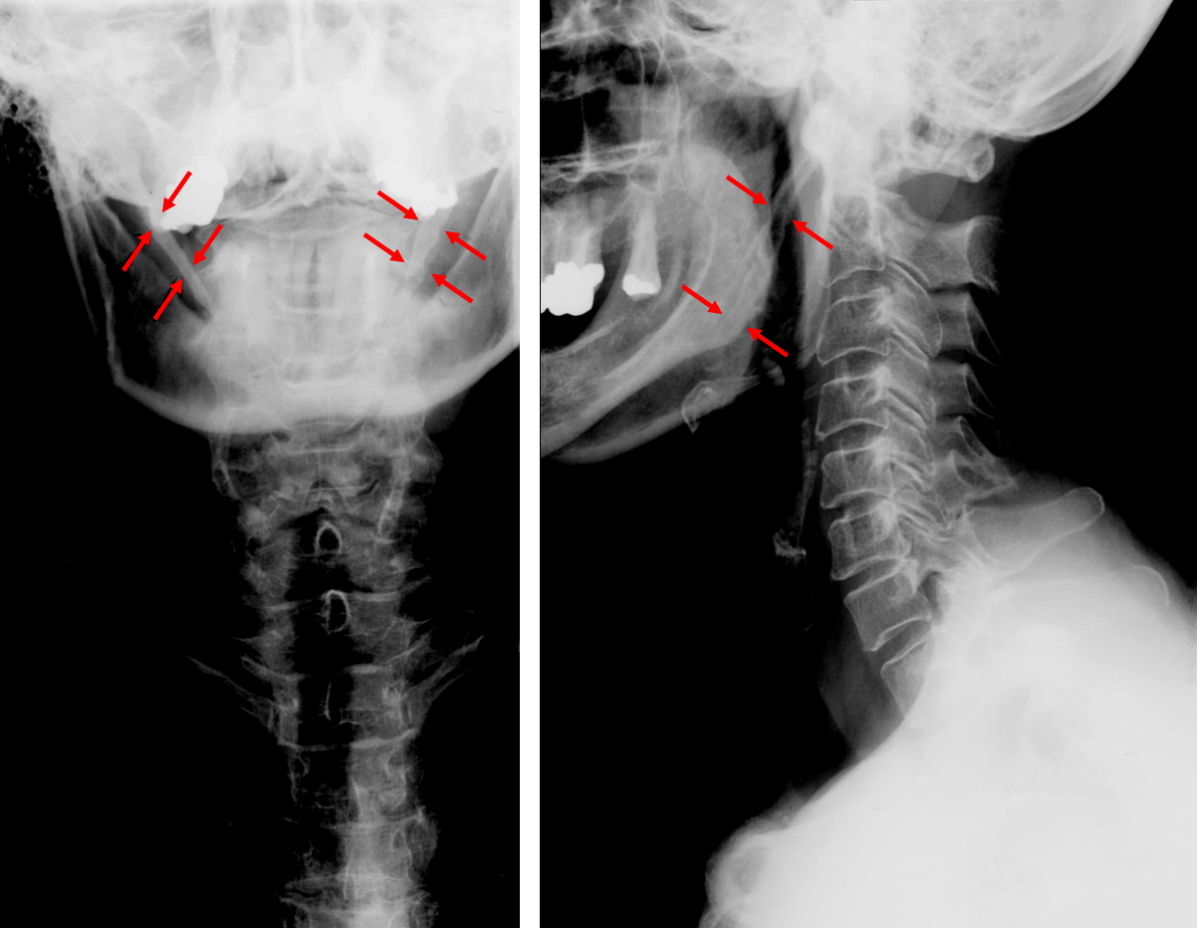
Radiographs of the vertebral spine: a-p and lateral view. Neither distinct malposition nor major degenerative changes of the cervical spine are recognizable. Formally and structurally inconspicuous cervical vertebral bodies and adnexa. But detection of a largely ossification of the ligamenta stylohyoidea on both sides. The patient's medical condition might be ascribed to a kerato-stylohyoidal syndrome.
